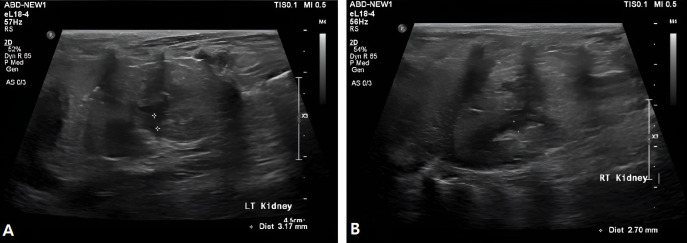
Ultrasonography of bilateral renal pelvis. (A) Ultrasonographic image of the left kidney demonstrating renal pelvic dilation measuring 3.17 mm. (B) Ultrasonographic image of the right kidney demonstrating renal pelvic dilation measuring 2.70 mm.
